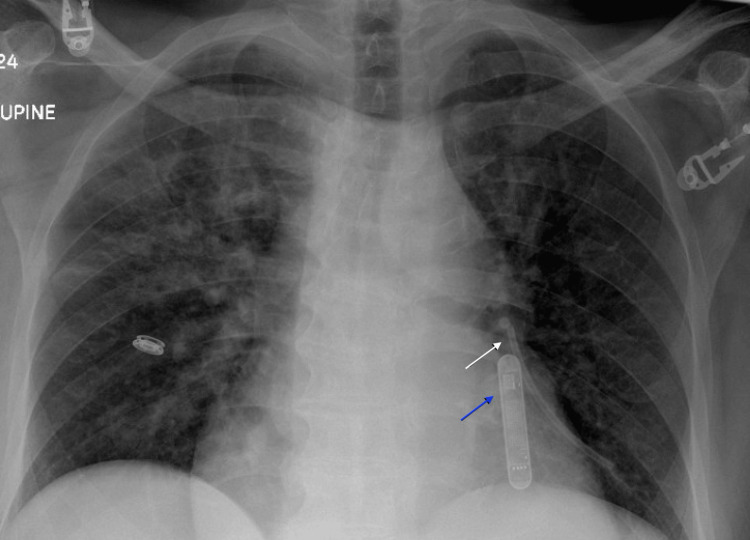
Postoperative chest X-ray Showing the interval removal of the catheter within the right subclavian vein. Presence of a retained catheter in the left pulmonary artery (marked by a white arrow). Preexisting loop recorder in the correct location (marked by a blue arrow).
